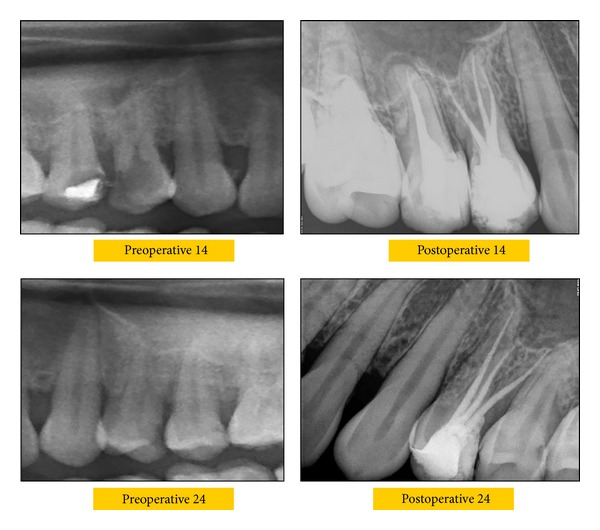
Preoperative and postoperative radiographs of 14 and 24 showing three roots.
